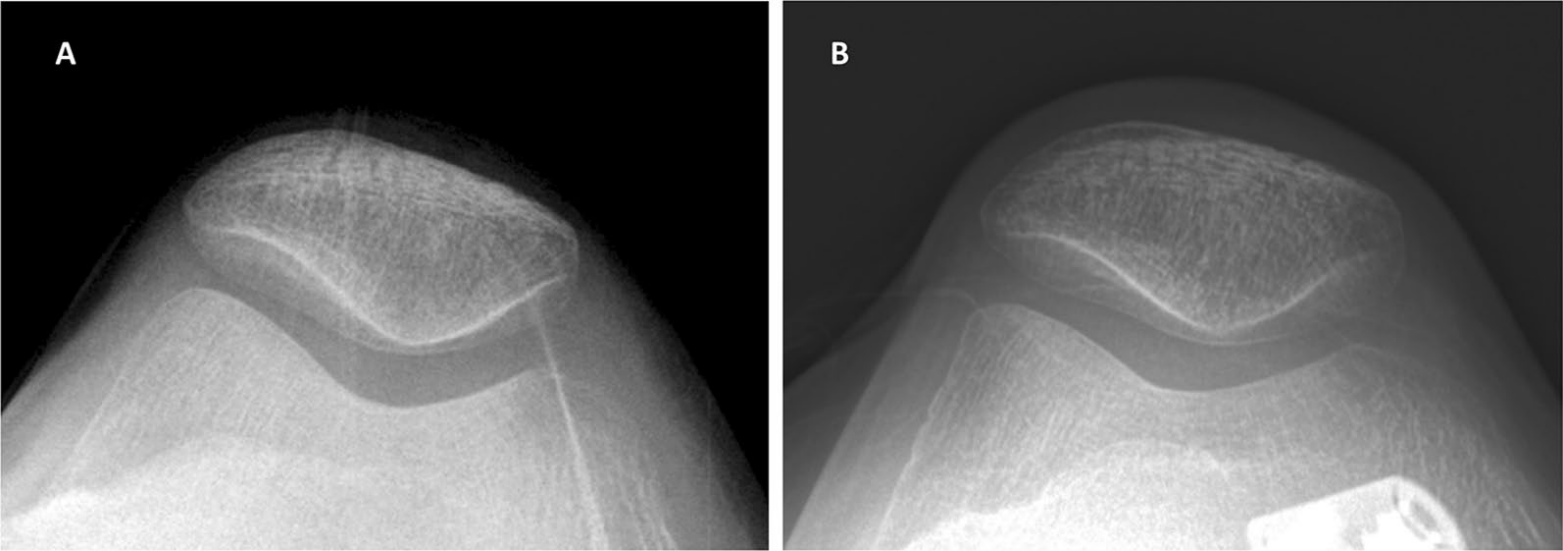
Merchant view radiographs of a 56-year-old woman (case 2). A Preoperative radiograph showing no patellofemoral joint osteoarthritis. B Follow-up radiograph 5 years after surgery showing no patellofemoral joint osteoarthritis
